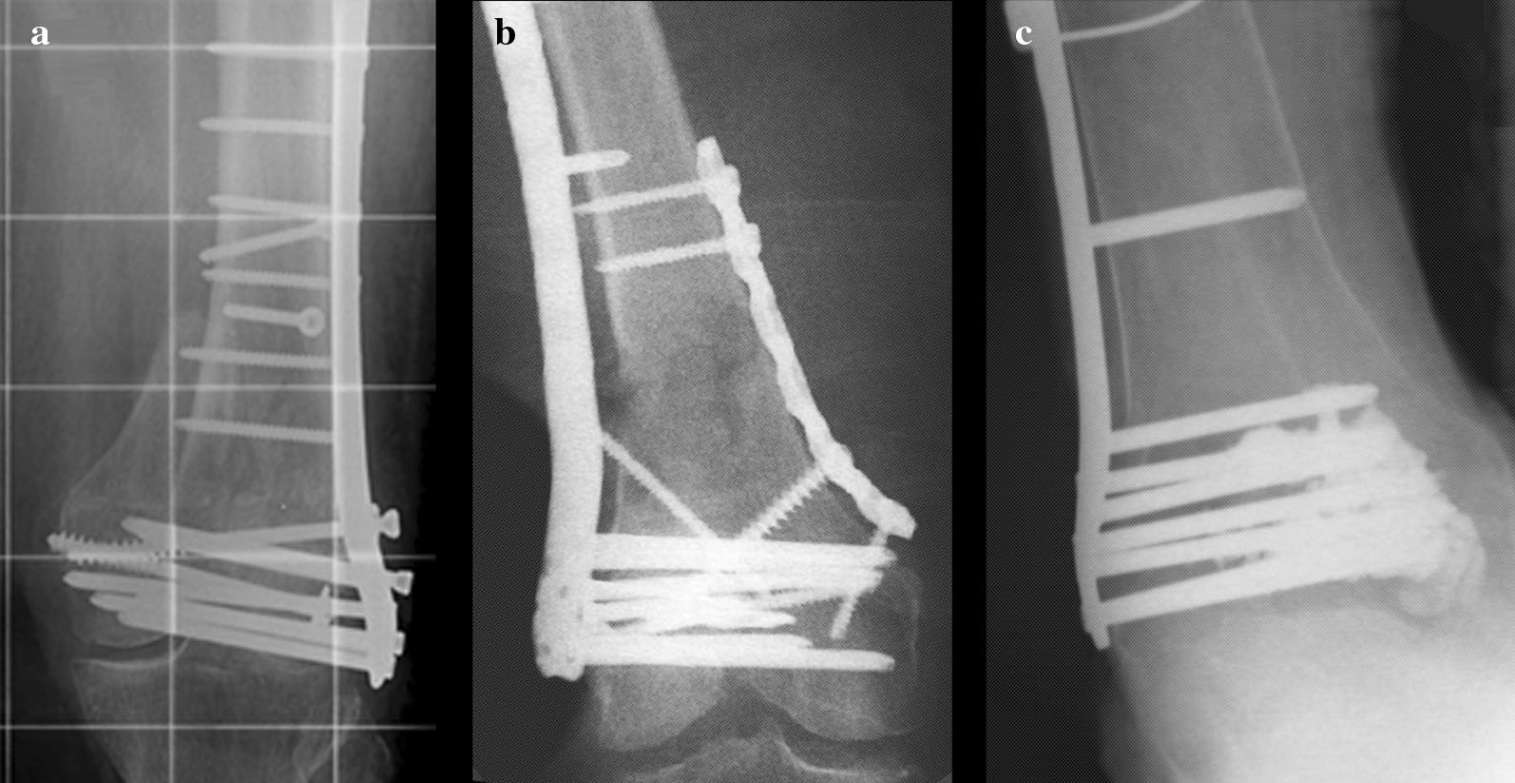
Distal femoral fracture cases. a X-ray of a distal femoral fracture after locked plating in anteroposterior projection; construct failure due to screw loosening and secondary loss of reduction is apparent. b X-ray of a distal femoral fracture treated with double plating to increase construct stability. c X-ray of a severe osteoporotic distal femoral fracture with locked plating and screw augmentation to enhance implant anchorage and construct stability
